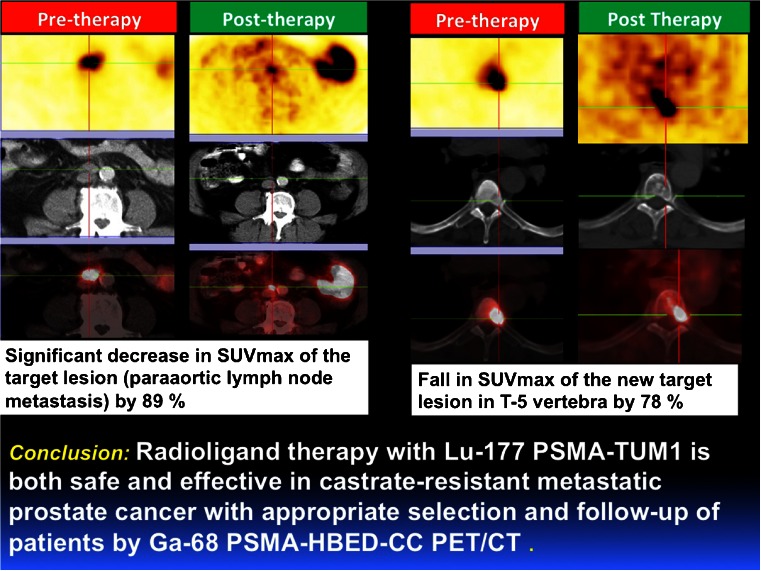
Antitumour efficacy of ^177^Lu-PSMA-TUM1 [48]
